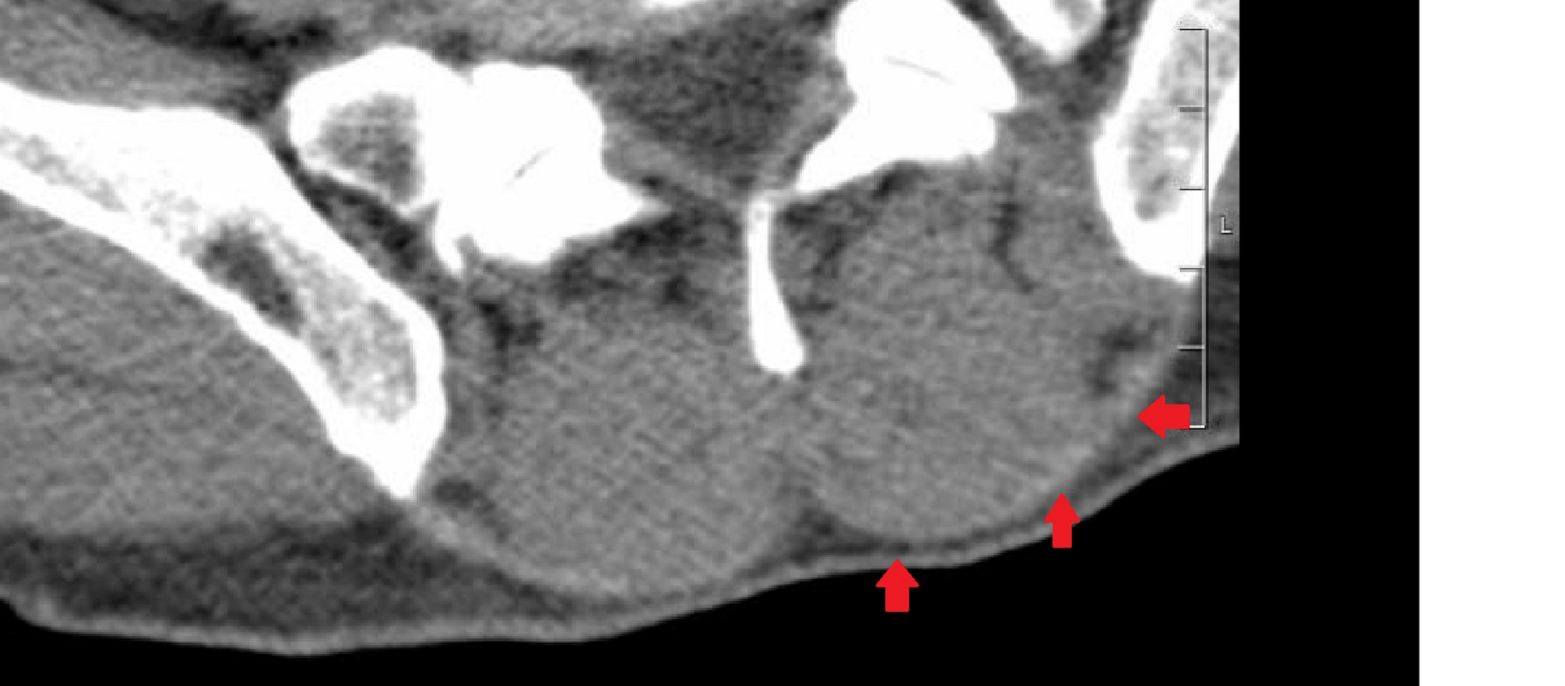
Computed tomography scan of the lumbar spine showing swelling of the left paraspinal compartment
